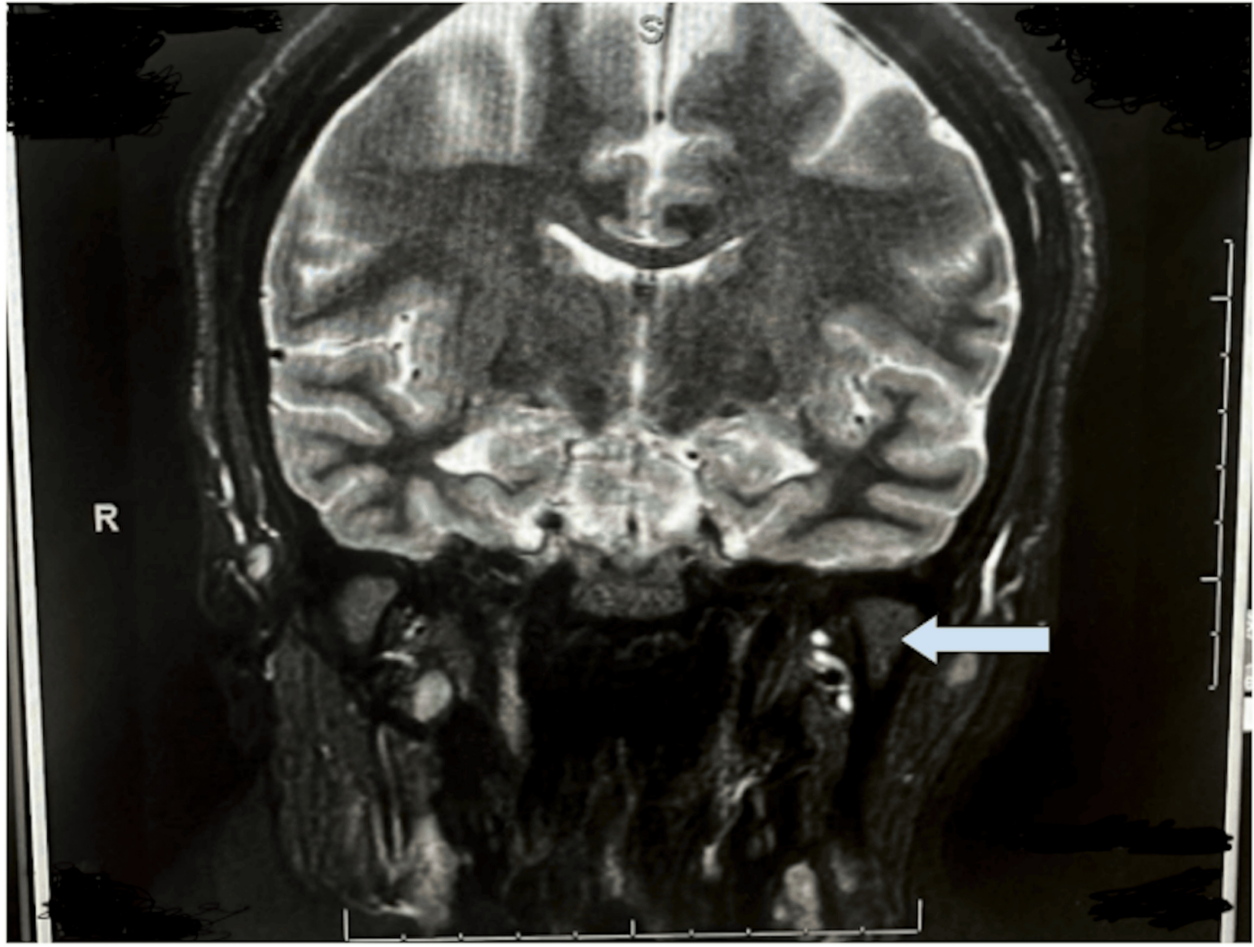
MRI of the orbits Blue arrow highlighting optic neuritis MRI: magnetic resonance imaging
